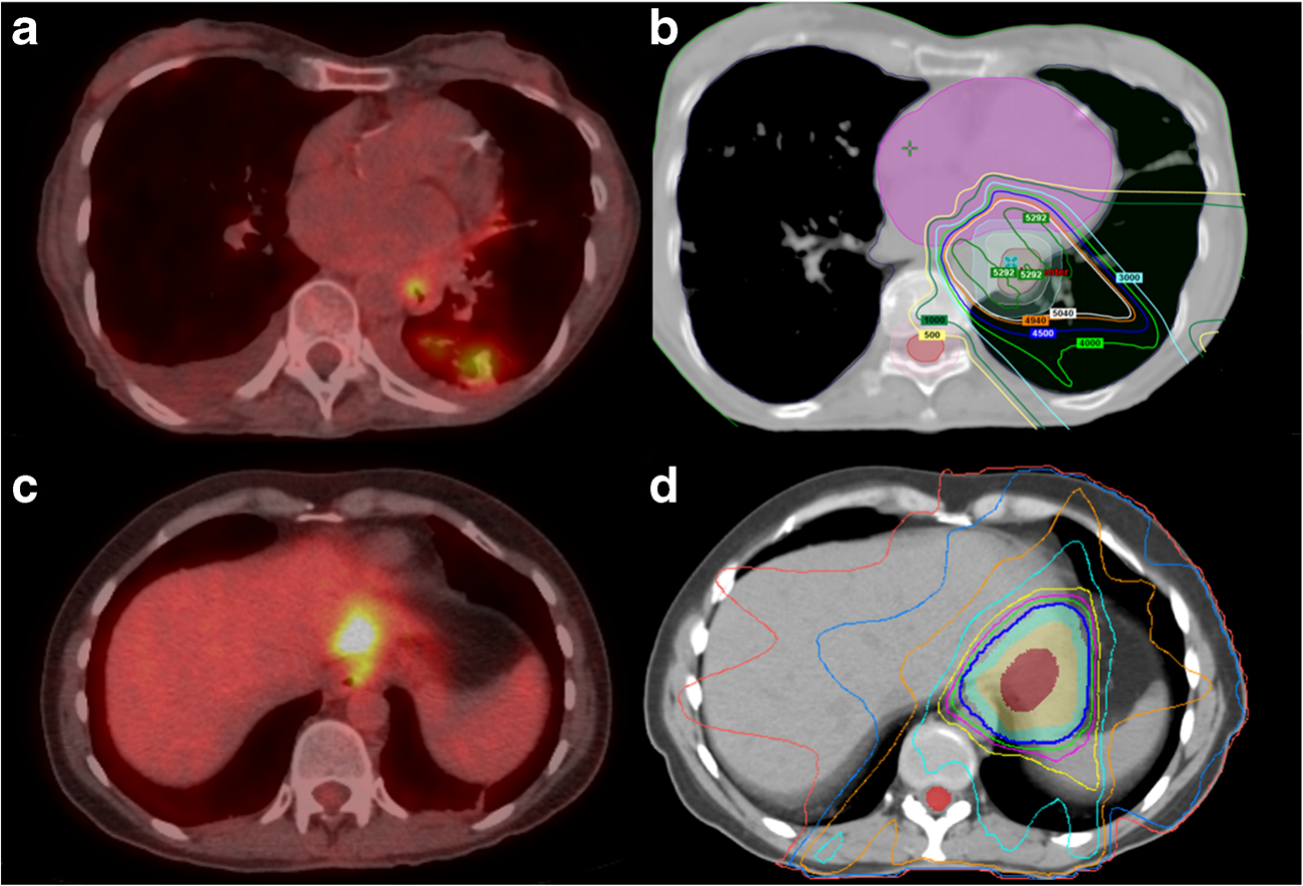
Examples of new non-malignant ^18^F-FDG avid lesions detected by ^18^F-FDG PET/CT restaging. (a): 78-year-old woman with squamous cell carcinoma of the esophagus treated with chemoradiation. The PET/CT image shows new opacities within the left lower lobe with corresponding areas of ^18^F-FDG activity. The new lesion was within the presumed radiation field (b) and the appearance was most compatible with radiation-induced pneumonitis (scan was regarded as ‘true negative’ for new metastatic disease). (c): 42-year-old female with adenocarcinoma of the distal esophagus who had undergone chemoradiotherapy. The PET/CT images show linear ^18^F-FDG accumulation within the lateral aspect of the left hepatic lobe. The new lesion was within the presumed radiation field (d) and was thought to be related to radiation therapy changes, which was confirmed with an MRI scan (scan was regarded as ‘false positive’ as additional imaging was required to exclude metastatic disease)
